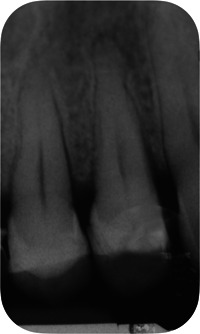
Baseline radiograph of the hopeless tooth
